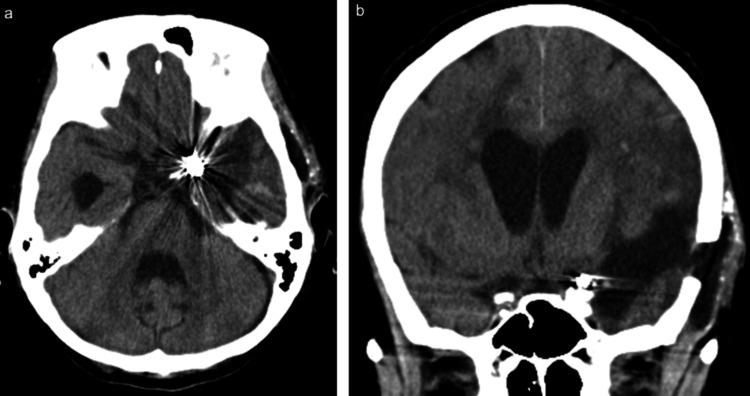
CT taken 20 days after endoscopic hematoma evacuation (a, b) Axial and coronal CT scans showing the post-surgical condition 20 days after hematoma evacuation.
